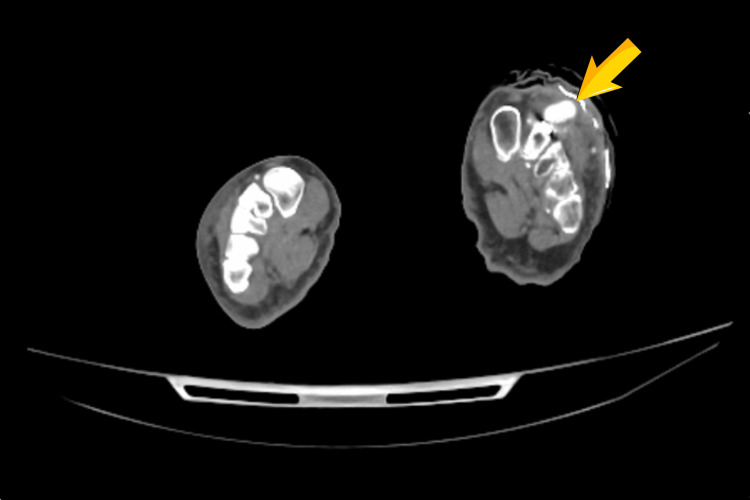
Axial CT angiography of the left foot demonstrating a well-defined contrast-filled outpouching (yellow arrow) arising from the dorsalis pedis artery at the level of the tarsometatarsal joints, consistent with a pseudoaneurysm.
